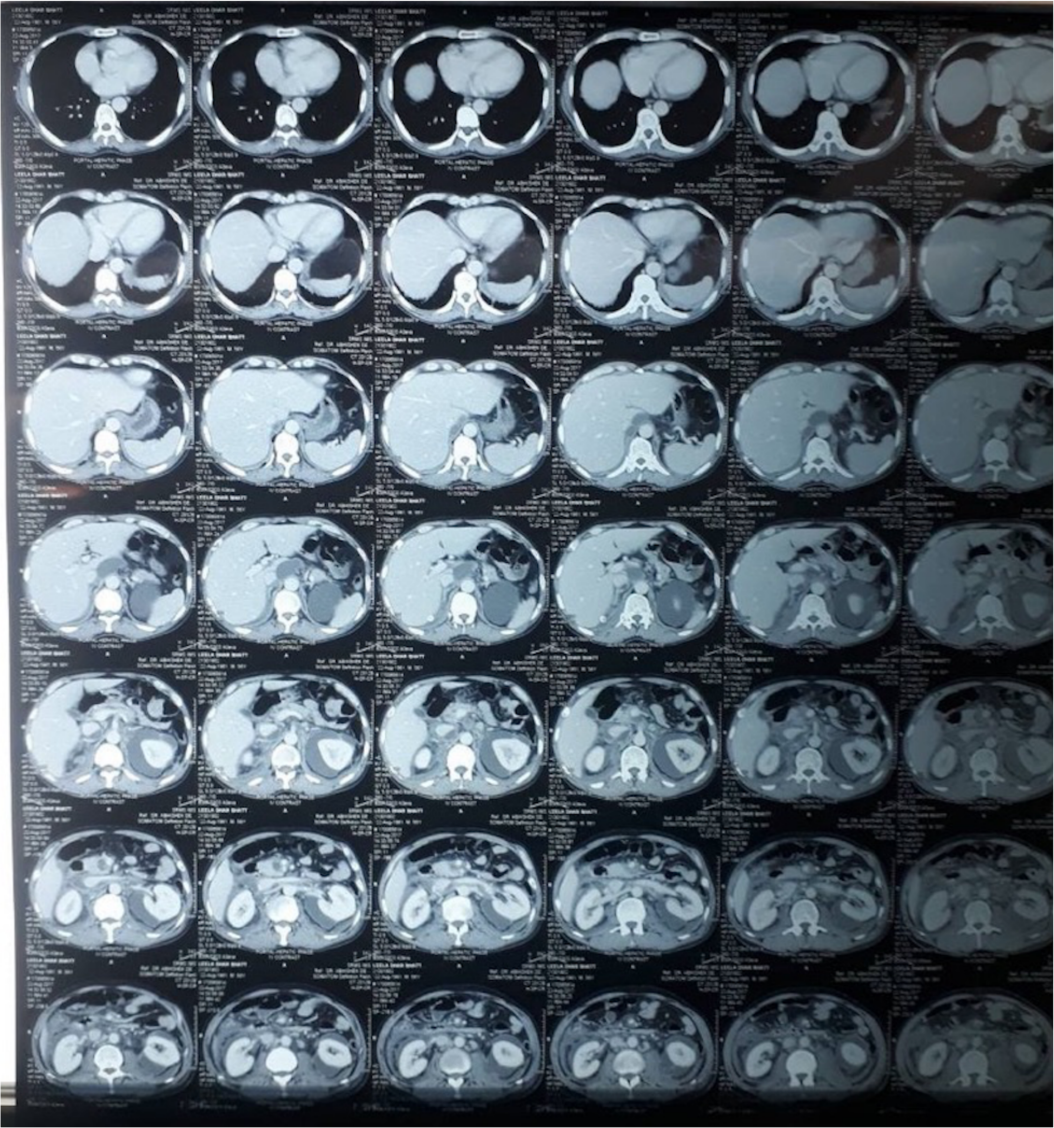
Computed tomography scan of the abdomen and pelvis showing decreased pancreatic bulk and small cystic area in uncinate process with adjacent peripancreatic and retroperitoneal collection extending to bilateral pararenal space
